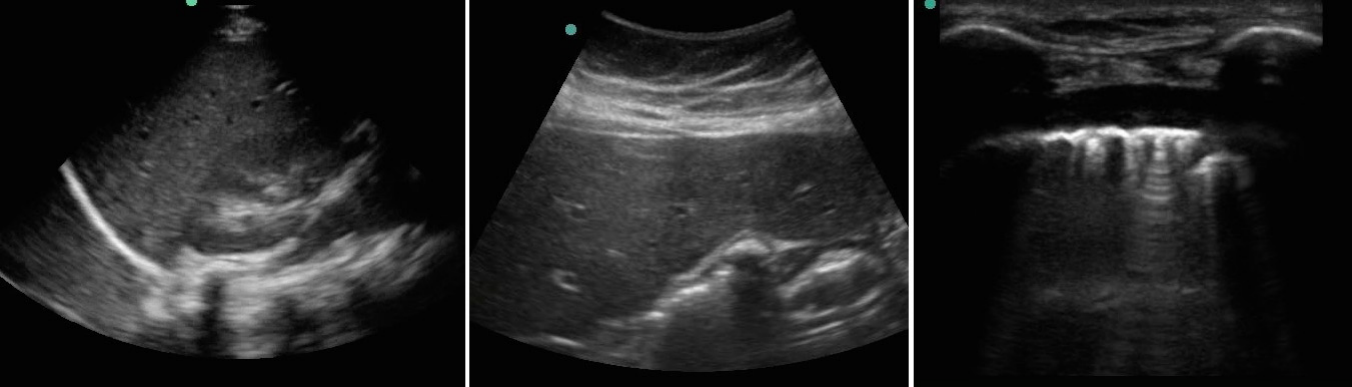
Ultrasound images taken with cassava gel. Images of the right upper quadrant FAST exam, positive gall stones and lung sliding taken with a Sonosite 180 ultrasound machine using cassava gel prototype.
